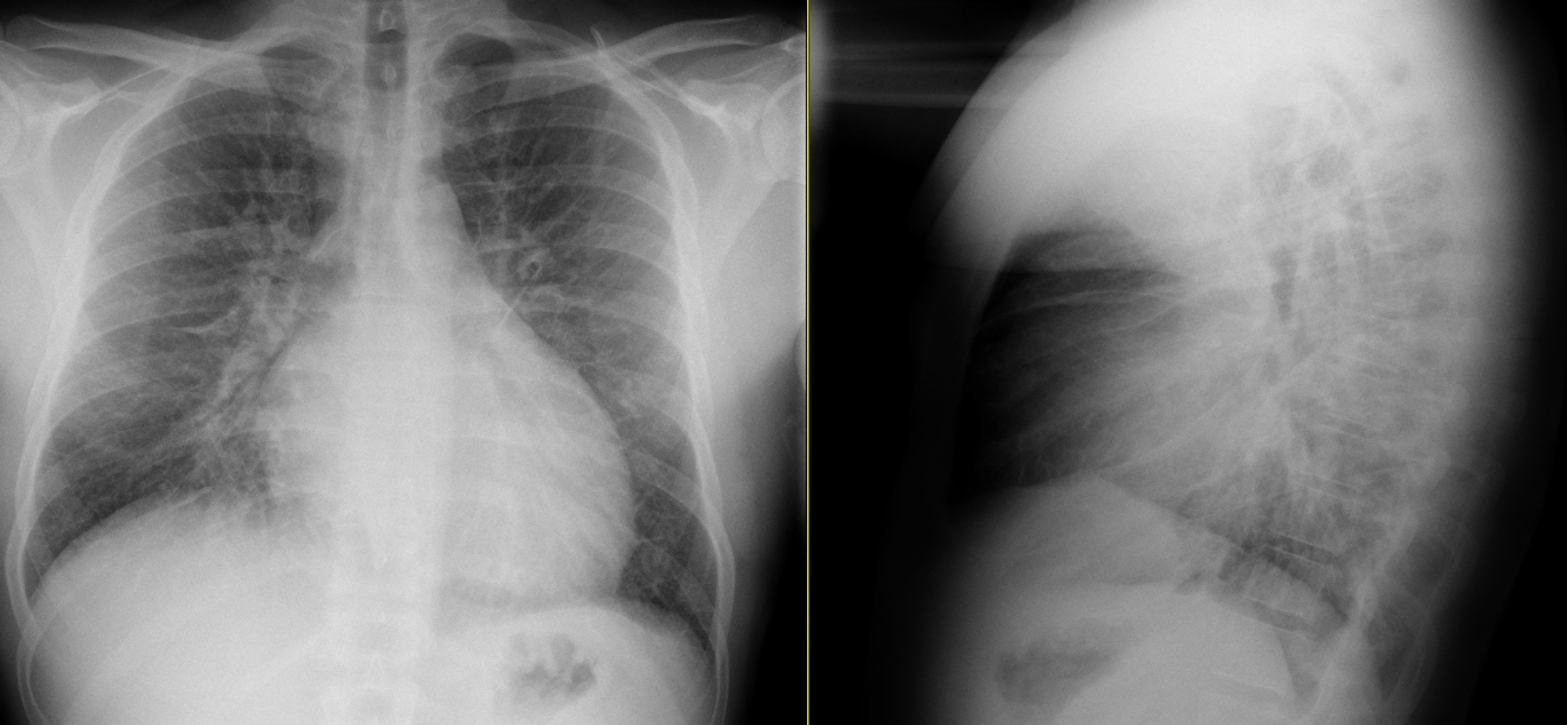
CXR showing prominent interstitial opacifications concerning for an infectious process versus pulmonary edema. Based on the lateral view, cardiac dimensions appeared within normal limits. No findings of obstructive lung disease were noted. CXR, chest X-ray
